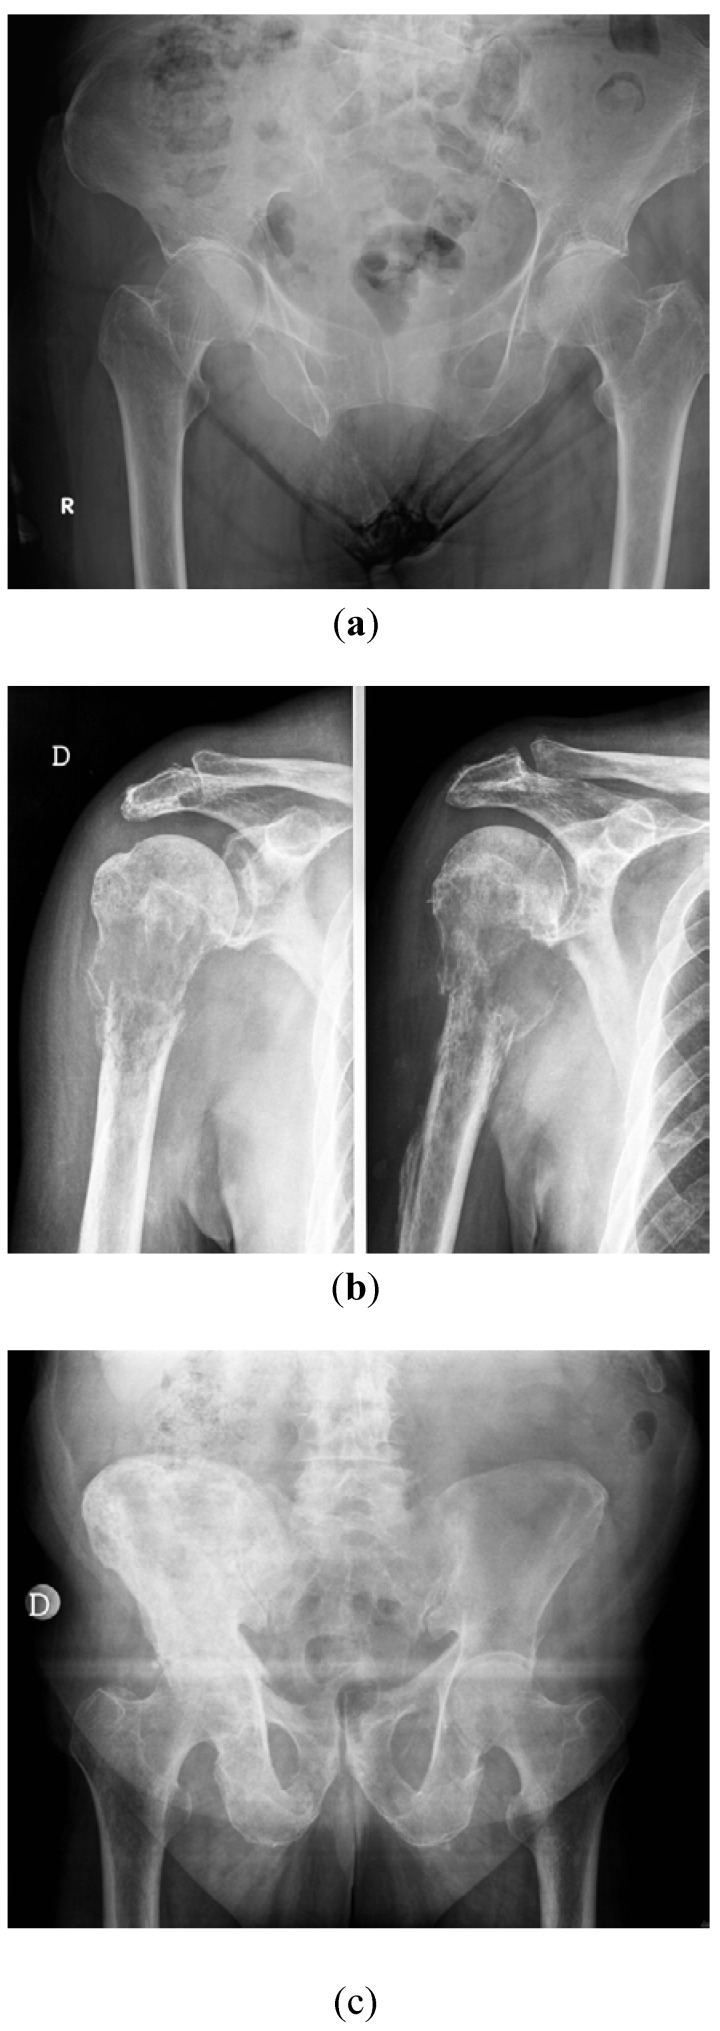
Standard X-ray examples of bone changes detectable in women with breast cancer: Osteoporosis of head femoral areas (a), pathological fracture of proximal third of the right humerus due to a large lytic lesion (b) and blastic bone infiltration of right hemipelvis (c).
